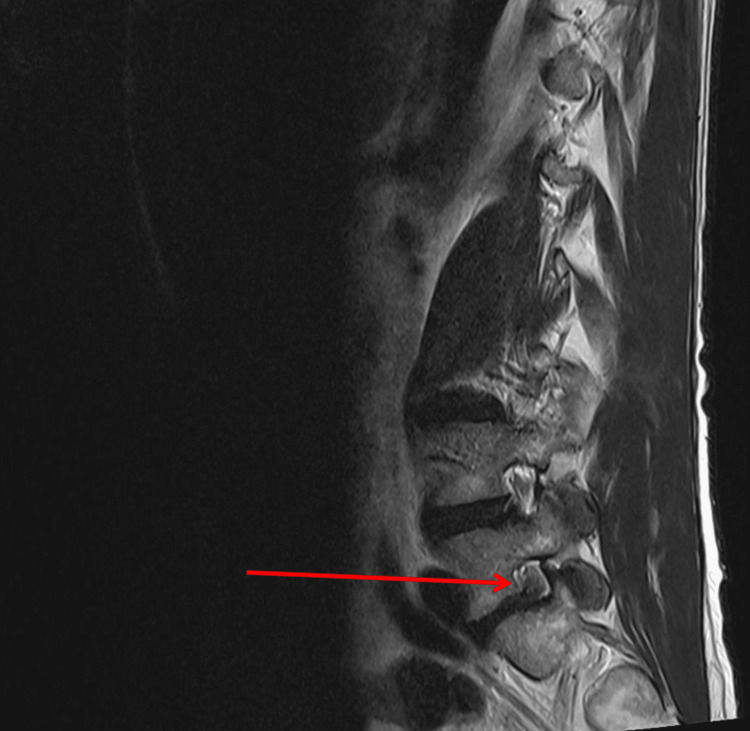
T2-weighted left parasagittal MRI of the lumbar spine. The red arrow depicts a duplicate left L5 exiting nerve root.
